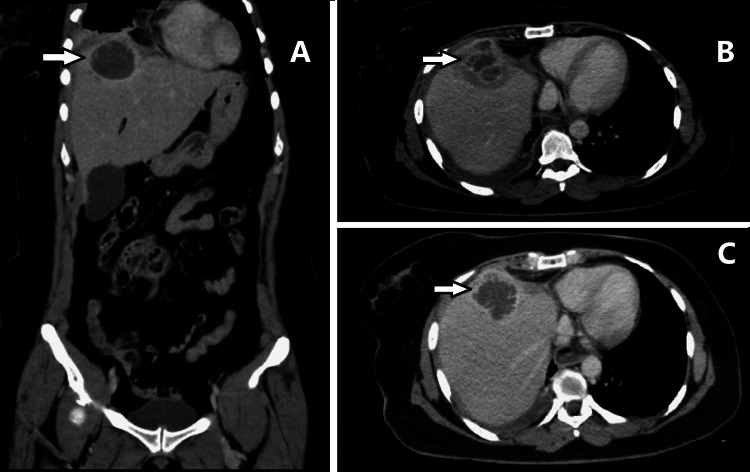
Thoracoabdominopelvic computed tomography scan showing a right hepatic abscess measuring 5 cm, with another contiguous component measuring 2 cm in the subphrenic region (white arrow)
